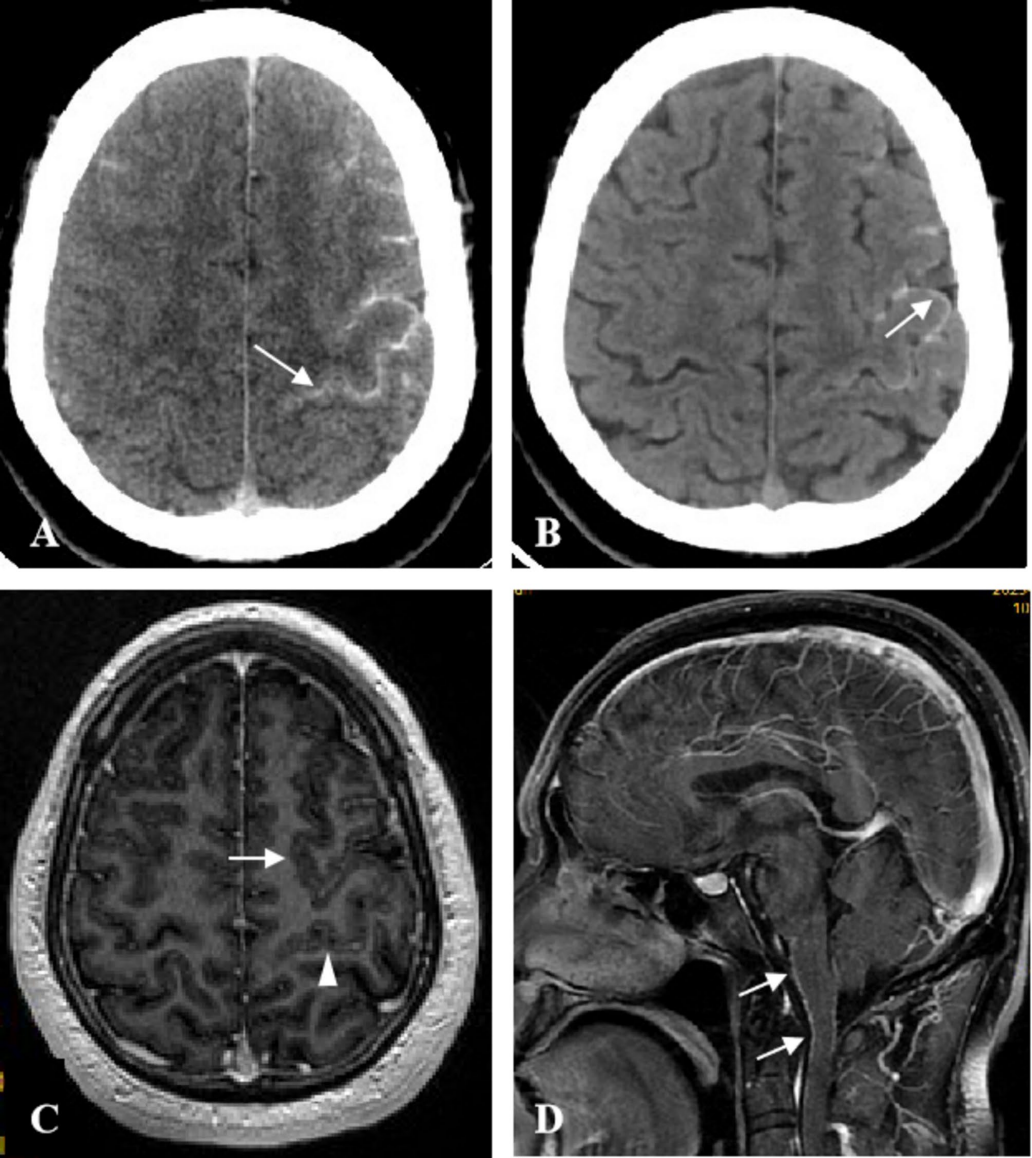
Post-interventional cerebral angiography CT images of the patient showing high density within certain cerebral sulci (A, indicated by arrows). Two days later, the high density within the cerebral sulci on CT imaging disappeared, and linear high density appeared in the leptomeninges (B, indicated by arrows). The following day, enhanced MRI of the head demonstrated linear enhancement of the leptomeninges and spinal membranes (C and D, indicated by arrows)
